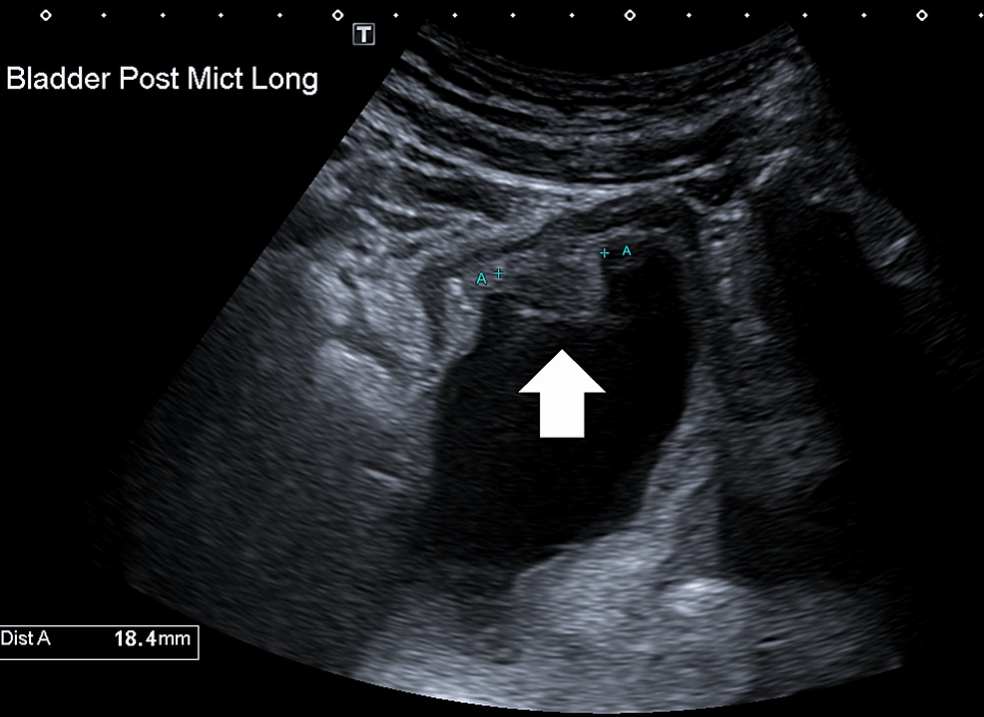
Ultrasound of the bladder: post-micturition, longitudinal view. An 18-mm mass was noted at the anterior bladder wall.
